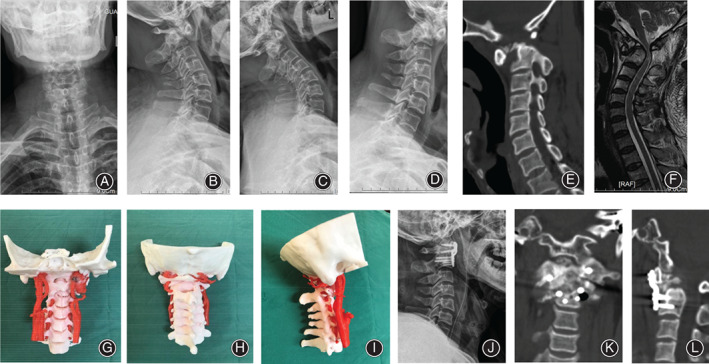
A 59‐year‐old male patient with irreducible atlantoaxial dislocation (IAAD) underwent transoral atlantoaxial reduction plate (TARP) surgery with the use of a three‐dimensional (3D) model. Preoperative images (A–F) show that the patient had atlantoaxial dislocation with compression of the spinal cord. The 3D model was made before the surgery, (G–I). Postoperative X‐ray and CT show atlantoaxial reduction after surgery (J–L).
